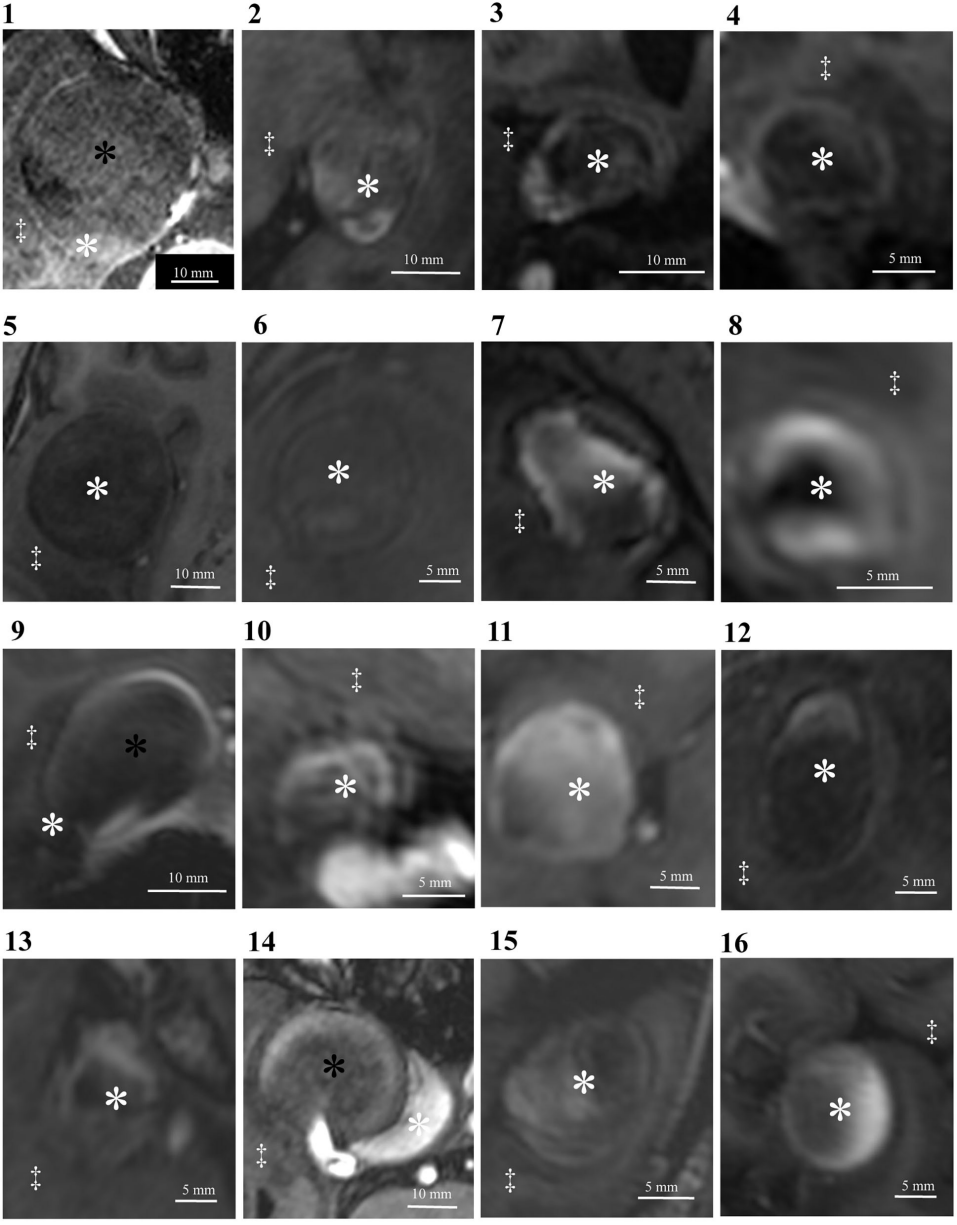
Delineation of thrombosed intracranial aneurysms using 7T MRI. Identical structures are marked in all subfigures as follows. White asterisks: intraluminal thrombus; black asterisks: aneurysm lumen; double daggers: brain parenchyma.
